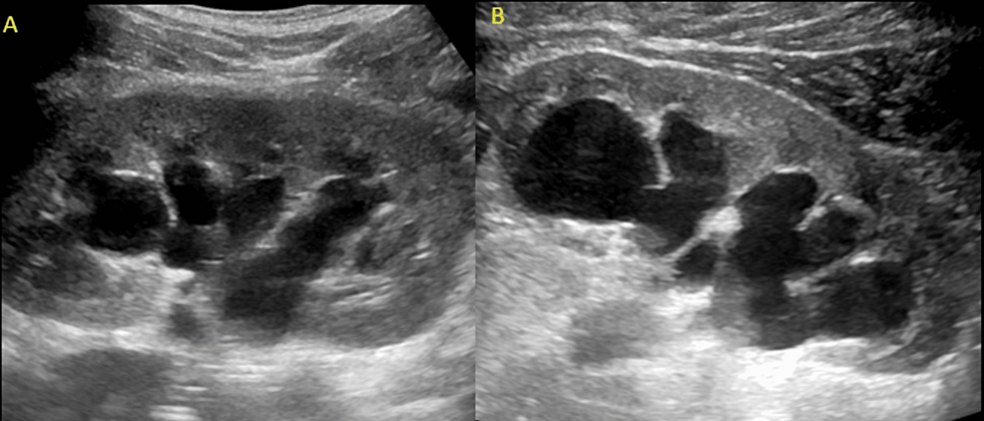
Grayscale ultrasound images of the kidneys Sonographic evaluation of the right (A) and left (B) kidneys showed bilateral hydronephrosis.
